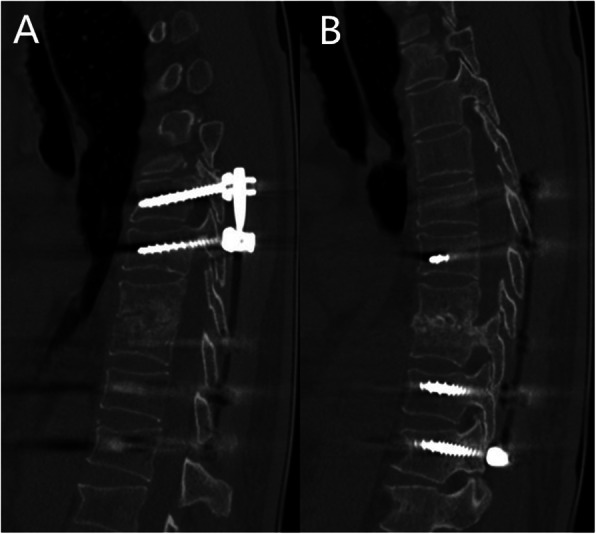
Postoperative CT imaging results after 4 months. a, b intervertebral fusion in T9-T10 was achieved
